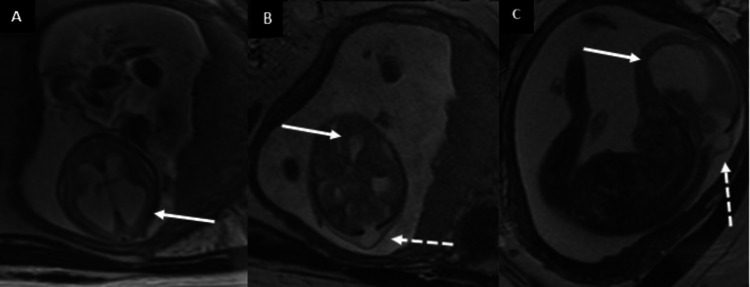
Fetal MRI: Chiari III Malformation Axial (A, B) and sagittal (C) T2-weighted sequences (A-C) demonstrate marked dilatation of the lateral ventricles (solid arrows). In images B and C, there is a cerebrospinal fluid (CSF) intensity outpouching through a midline occipital bone defect (dashed arrows).
